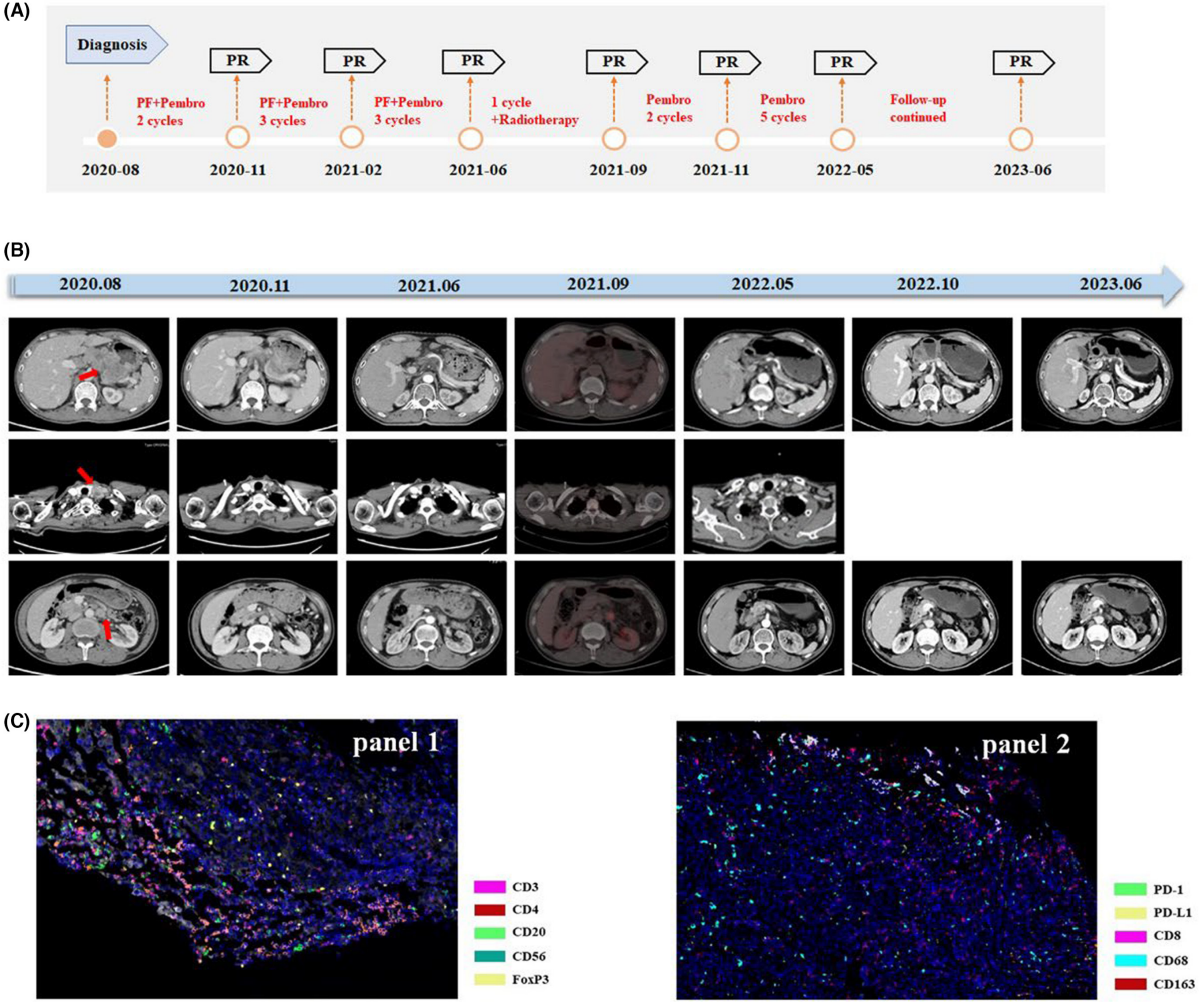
Treatment details, imaging radiographic findings, and mIF analysis of the case. (A). Timeline scheme of this patient. (B) Representative images of the major clinical event since diagnosis. (C) Immune cell distribution of this patient. Representative images for Panel 1. CD3, CD4, FOXP3, CD20, and CD56. Panel 2. CD8, PD‐1, PD‐L1, CD68, and CD163 expression in the TME. ×200 magnification.
